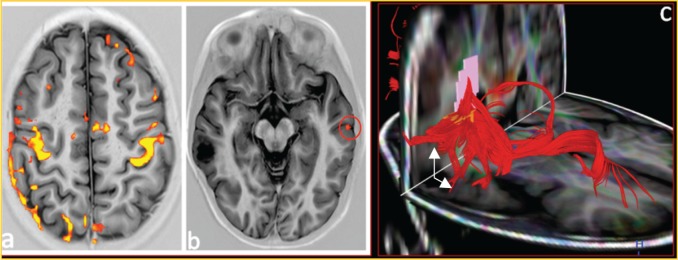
(a) Functional BOLD–MRI shows areas of motor cortex and somato-sensory activation during the bilateral motor palmar paradigm. (b) Areas of activation for the first left temporal girus with participation of the ipsilateral frontopercular region during the paradigm of processing words in silence observing areas of activation in the contra-lateral hemisphere (red circle). (C) 32 directions tractography, shows association fibres of association, demonstrating moved fascicle arcuate (white arrows).
